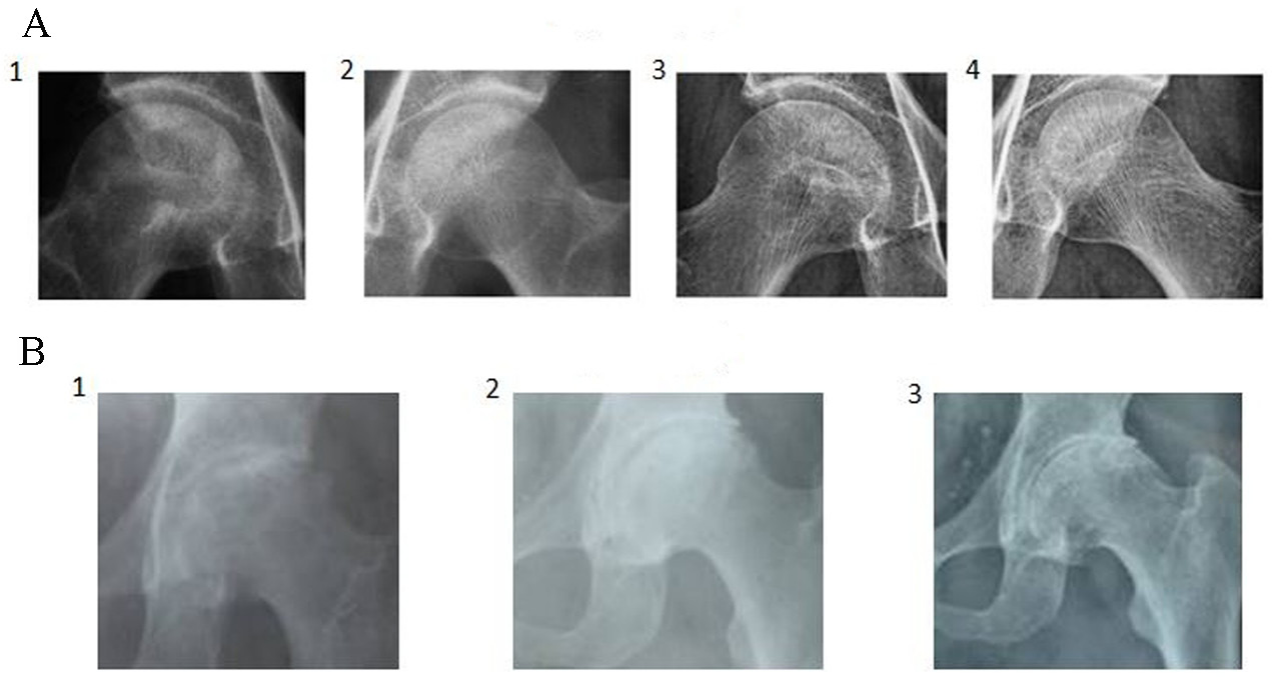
Representative case of osteonecrosis of the femoral head (ONFH) by X-ray analysis (A) 52-year-old male patient, alcoholic. 1 and 2: The density of the femoral head was not uniform and bone trabeculae disappeared, especially on the right side. 3 and 4: After treatment with the bone-strengthening pill (BSP), the pathological radiographic findings disappeared and the patient resumed work. (B) 58-year-old male patient, no clear etiology was determined. 1: The outline of the femoral head was disrupted with the disappearance of the joint space and loss of bone trabeculae. 2: After treatment with BSP, the surfaces of the femoral head became smooth with joint space restored and clear margins of bone trabeculae. The patient started light work after treatment. 3: A 16-year follow-up showed a smooth surface of femoral head, clear joint space, and bone trabeculae.
